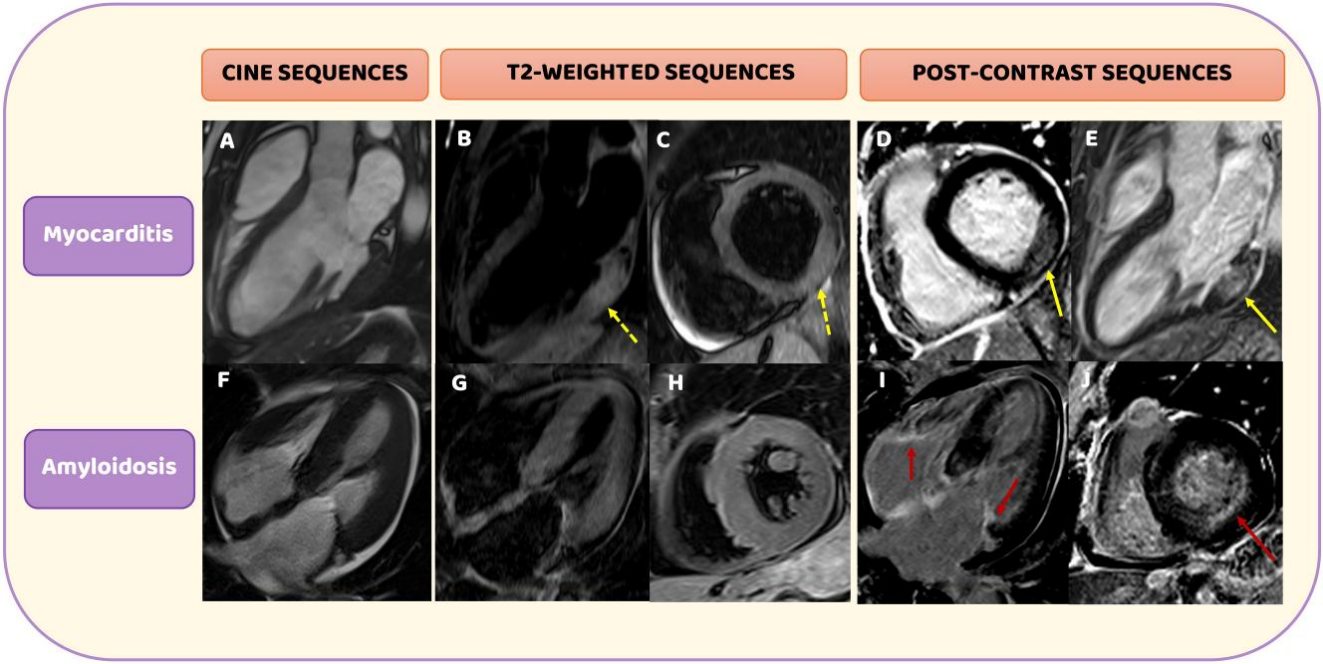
Cardiac magnetic resonance cine, T2-weighted, and post-contrast sequences in patients with myocarditis and cardiac amyloidosis. (*A–E*) A representative case of a 31-year-old male patient with myocarditis and left ventricular hypertrophy (*A*), demonstrating myocardial oedema on T2-weighted images (*B–C*, dotted yellow arrows) and subepicardial late gadolinium enhancement in the basal inferolateral wall on post-contrast sequences (*D–E*, yellow arrows). (*F–J*) A representative case of a 72-year-old female patient with cardiac amyloidosis and concentric wall thickening (*F*). No myocardial oedema is observed on T2-weighted sequences (*G–H*). Post-contrast images reveal diffuse subendocardial late gadolinium enhancement involving the basal inferolateral wall (*J*, red arrow) and extending to the atrioventricular valves and atrial walls (*I*, red arrows).
